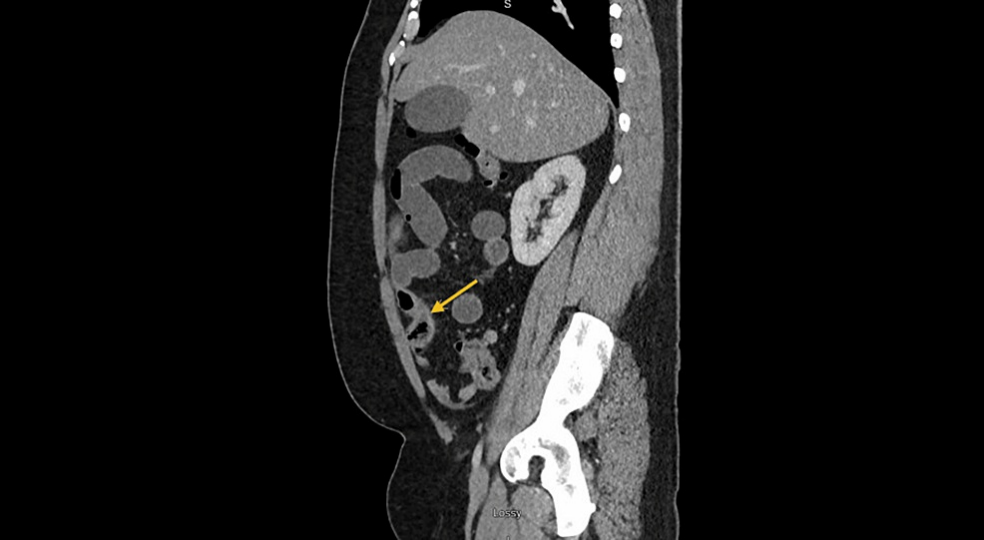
Abdominal CT scan showing dilated proximal bowel with decompression and apparent transition point (yellow arrow) in the distal small bowel
